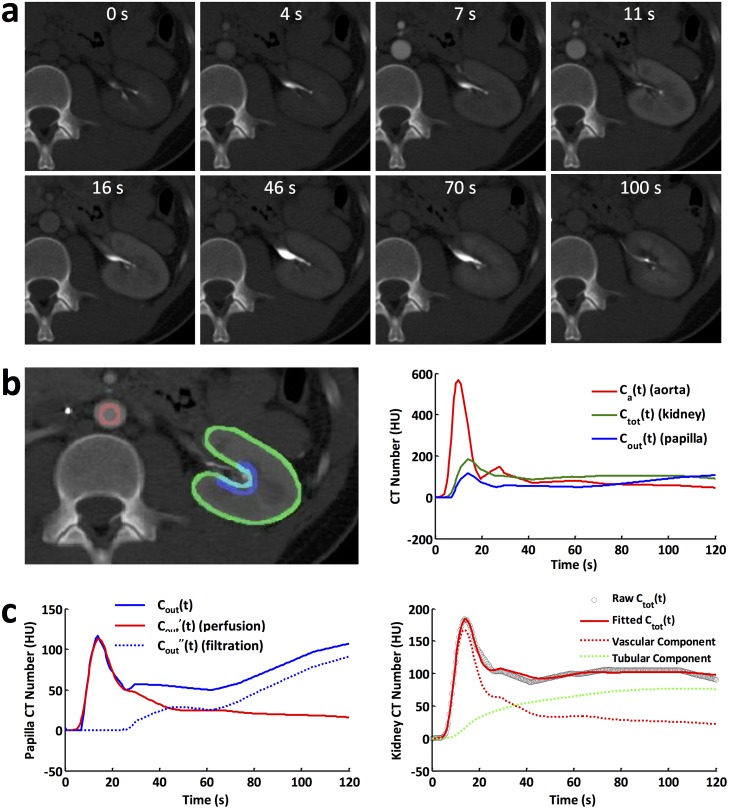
DCE-CT and model fitting. (a) Representative CT images acquired at baseline, and 4, 7, 11, 16, 46, 70, 100 sec post-contrast injection. (b) Three ROIs (left) manually traced on abdominal aorta (red), renal parenchyma (green), and papilla (blue), and their respective time attenuation curves (right). (c) Time attenuation curve in renal papilla decomposed into perfusion and filtration components (left), and model fitting to the time attenuation curve in the renal parenchyma, as well as the fitted vascular and tubular components.
